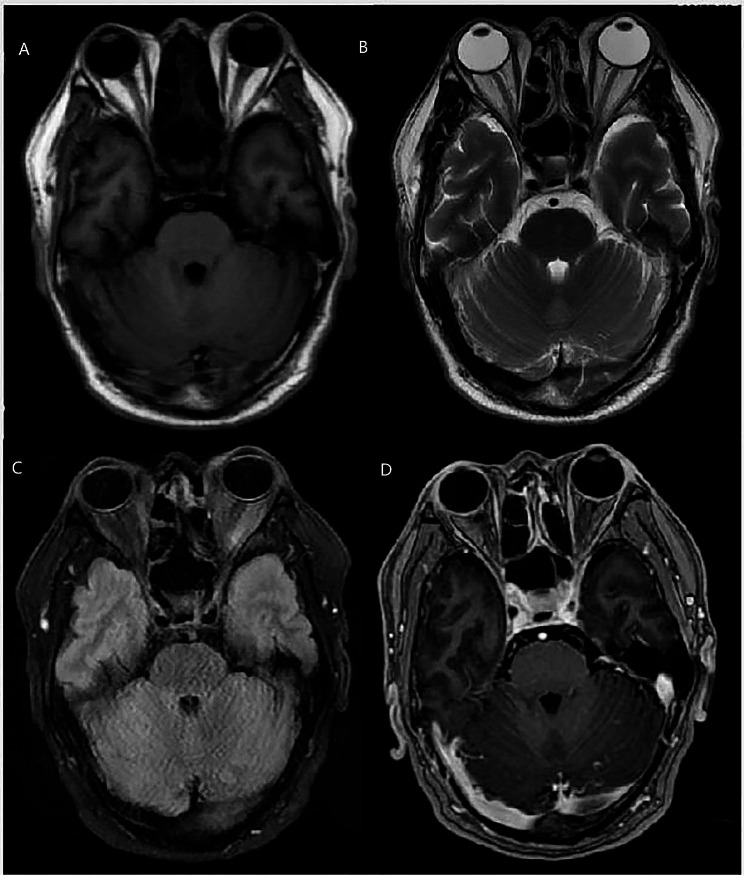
Head MRI with contrast showed no abnormalities
